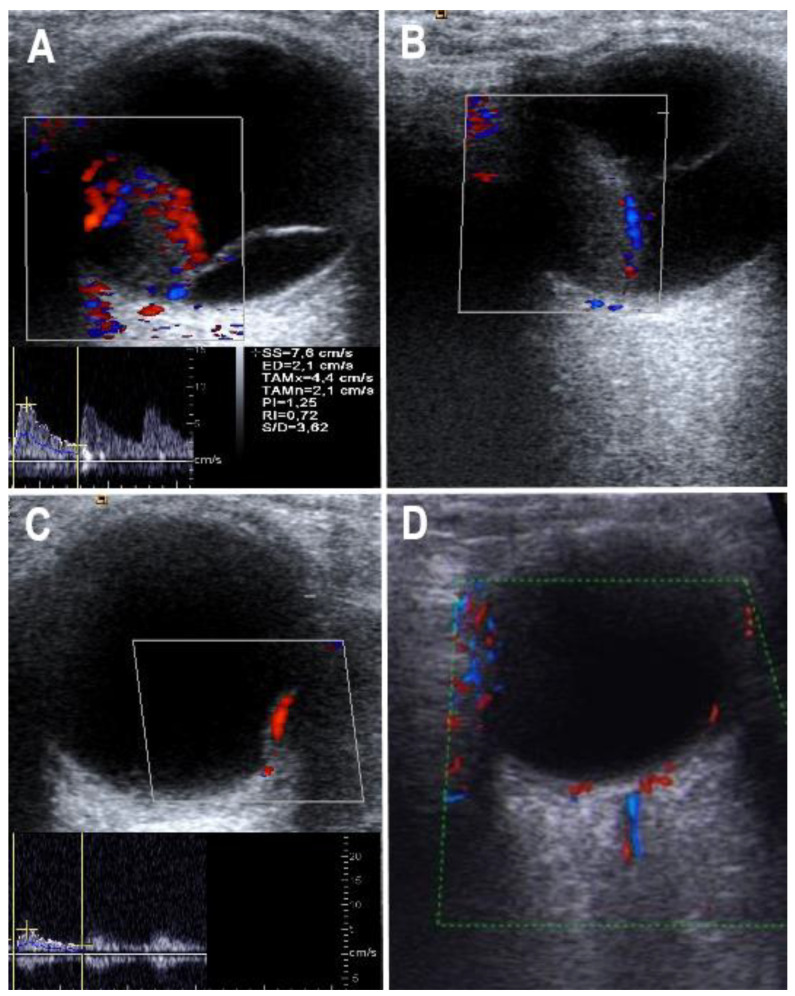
Color Doppler flow imaging of choroidal melanoma before and after therapy. (A) Patient with a large choroidal melanoma at inferior nasal location of left eye. Note the high degree of intra-tumor vascularization (level 3). The insert in the lower part shows the CDFI flow curve and indices of a prominent intra-tumor artery. (B) Choroidal melanoma 3 months after CyberKnife^®^ therapy in the same patient as shown in (A). Note the complete loss of intra-tumor vascularization (level 0), with visibility only of sub-retinal vessels. (C) Patient with a small choroidal melanoma at temporal location of right eye. Note the prominent singular intra-tumor artery (vascularization level 2). The insert in the lower part shows the CDFI flow curve of the intra-tumor artery. (D) Choroidal melanoma 12 months after CyberKnife^®^ therapy in the same patient as shown in (C). Note the nearly complete loss of intra-tumor vascularization (level 1), with visibility only of a low-flow intra-tumor artery.
